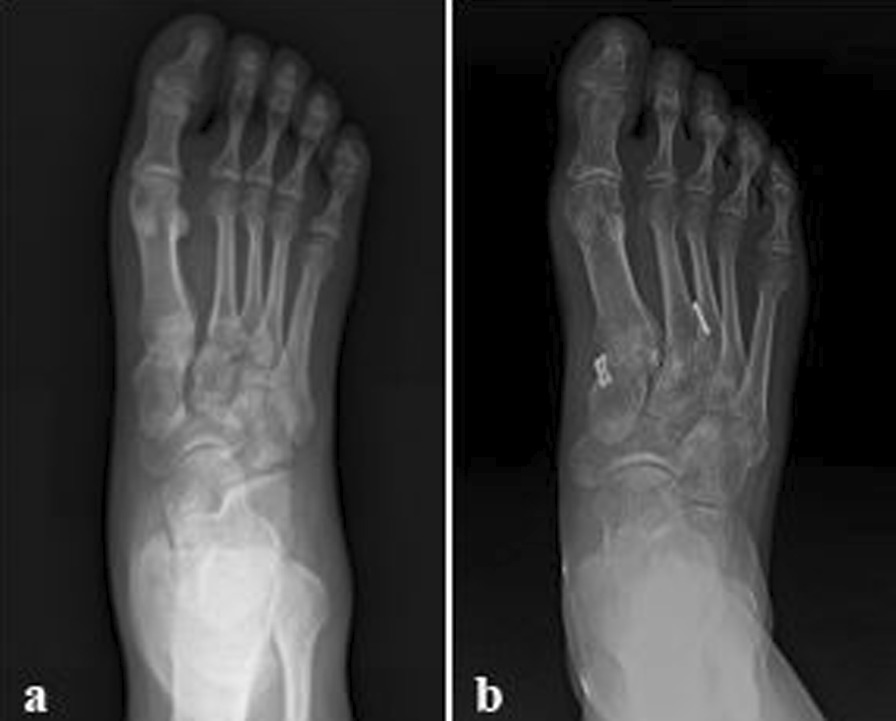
a, b Anteroposterior (AP) foot radiograph of early postoperative and the last follow up
